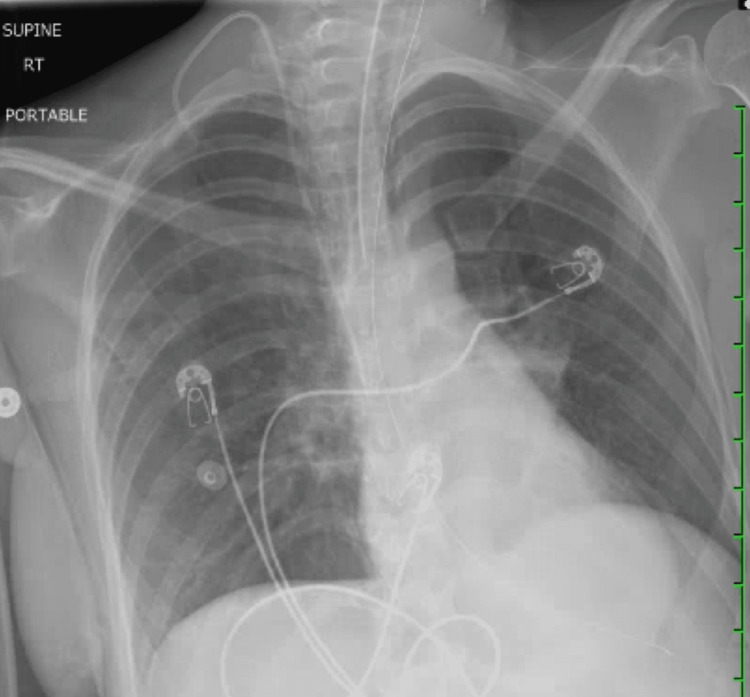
Chest radiograph demonstrating endotracheal tube.
